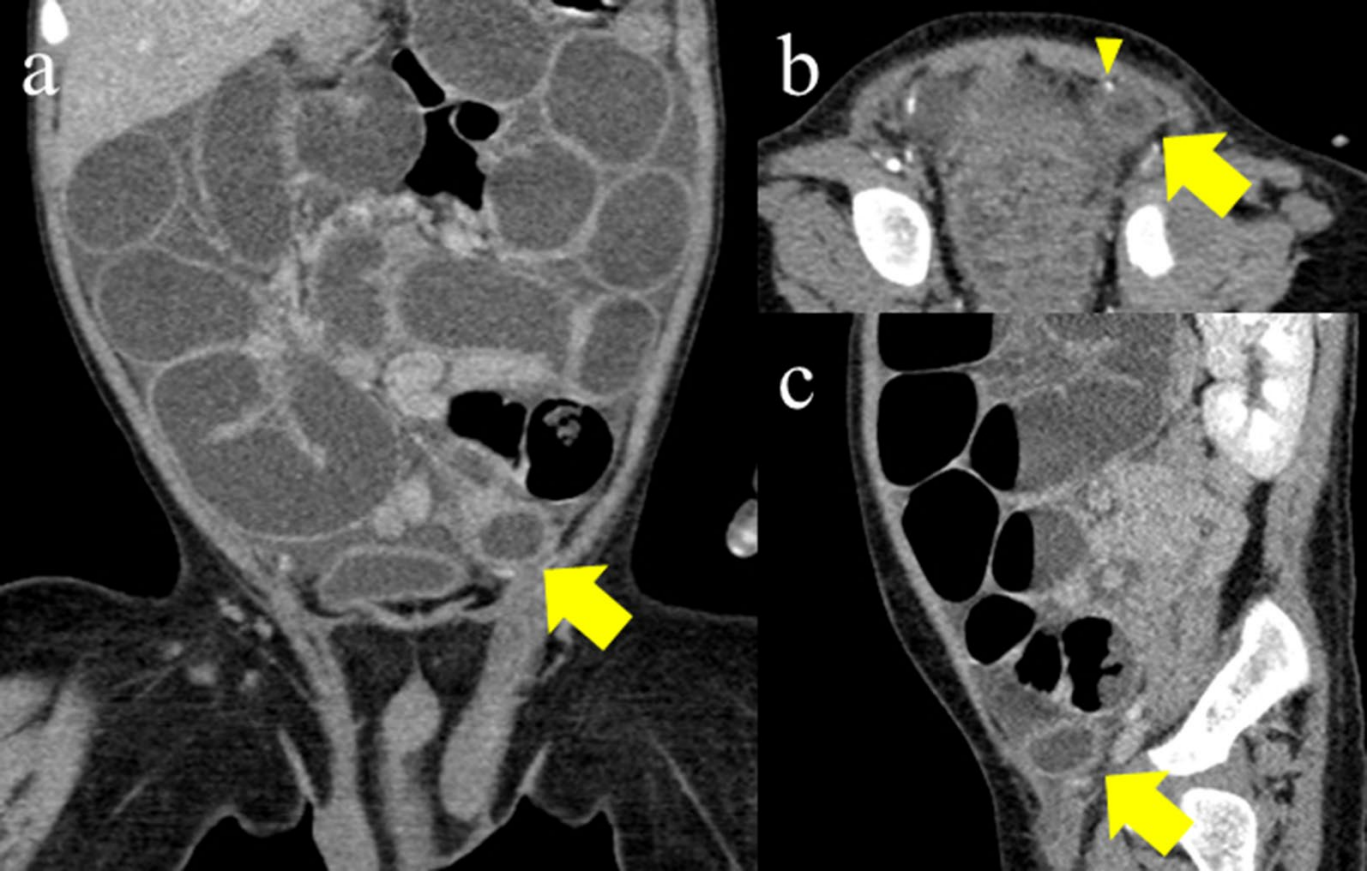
Enhanced CT findings. Closed loop in the left groin (yellow arrow). a Coronal section. b Axial section and inferior gastric artery (yellow arrowhead). c Sagittal section
